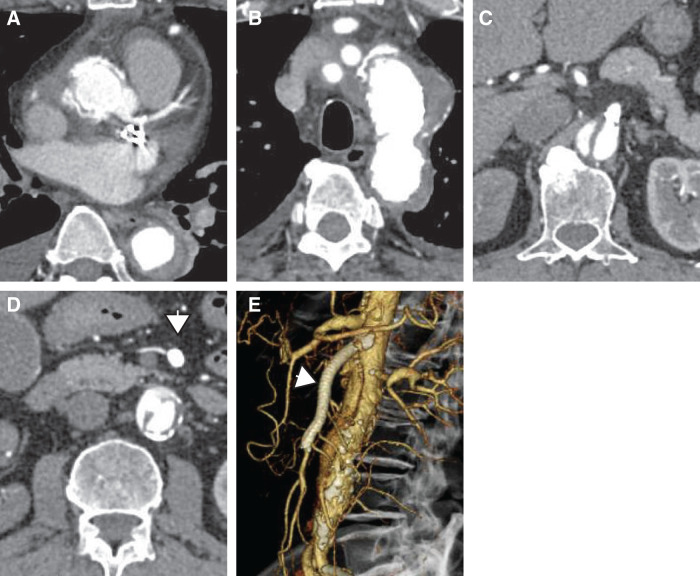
CT findings following TAR with FET. (A) The ascending aorta has been replaced with a prosthetic graft. (B) The saccular aneurysm in the distal arch shows complete thrombosis. (C) Blood flow to the SMA origin is restored through the true lumen. (D, E) The main trunk of the SMA demonstrates patent flow (white arrowhead). FET, frozen elephant trunk; SMA, superior mesenteric artery; TAR, total arch replacement
